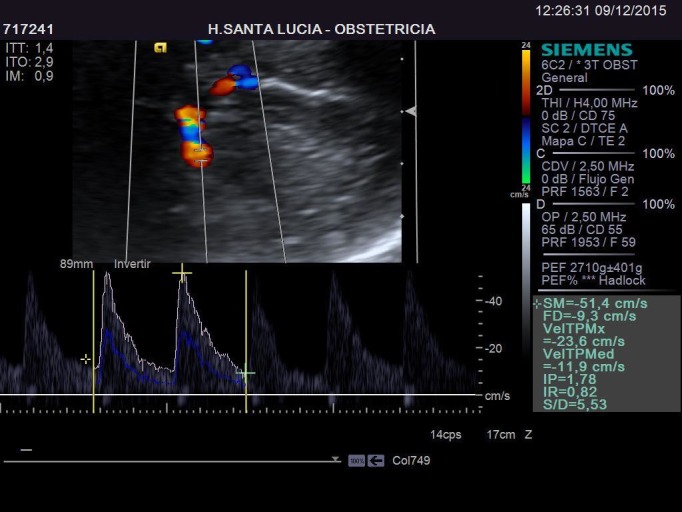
Middle Cerebral Artery Doppler in Week 35
